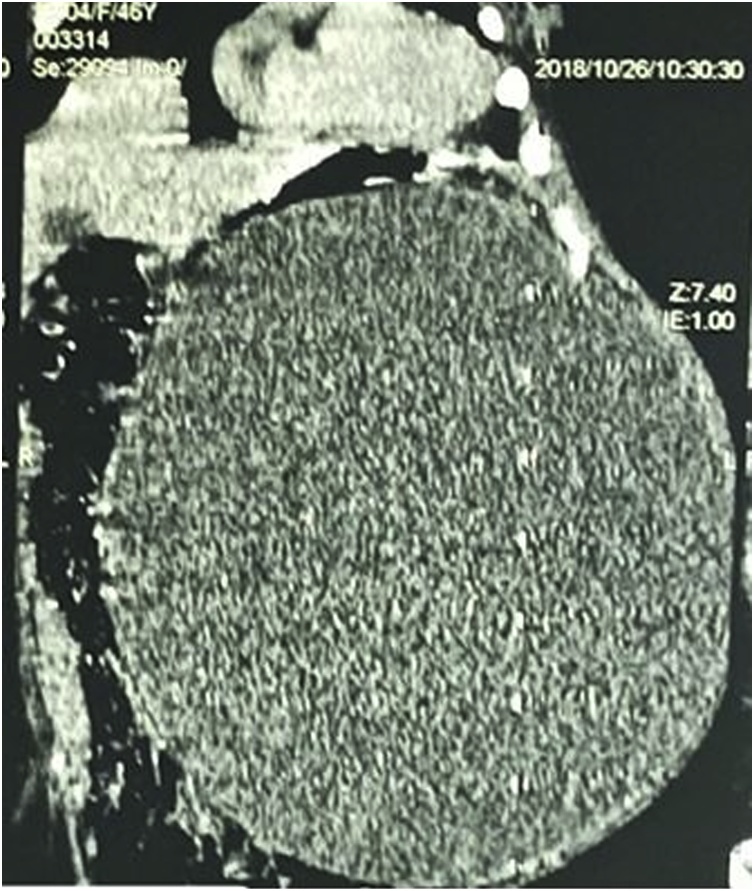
Abdominal CT scan showing a homogenous mesenteric abdomino-pelvic mesenteric cyst lateralise to the left, measuring 304 × 317 × 240mm.
